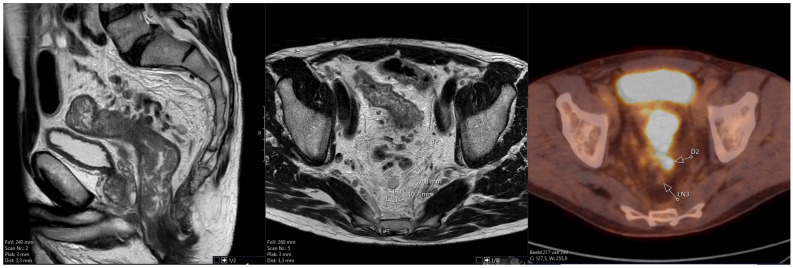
Example of the anonymized combined MRI and F18-FDG PET/CT layout used in this study. The example shows a tumor deposit (designated D2) and a lymph node (designated LN3). Only the primary tumor is depicted on the sagittal image.
